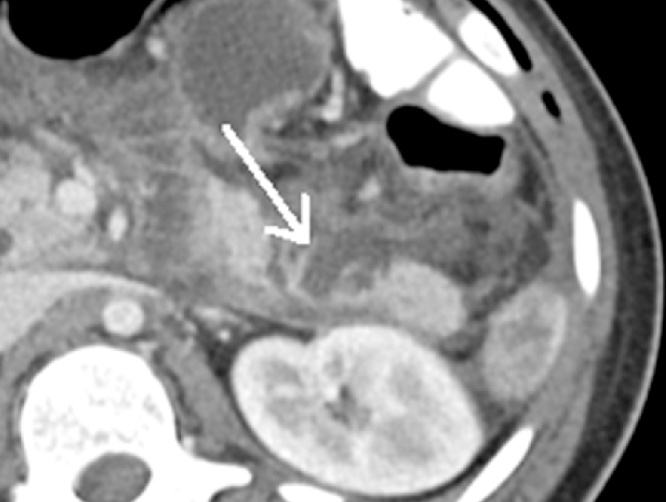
Patient 1. CT on second postop day. Lack of perfusion (arrow) of a distal segment of the pancreatic body.
